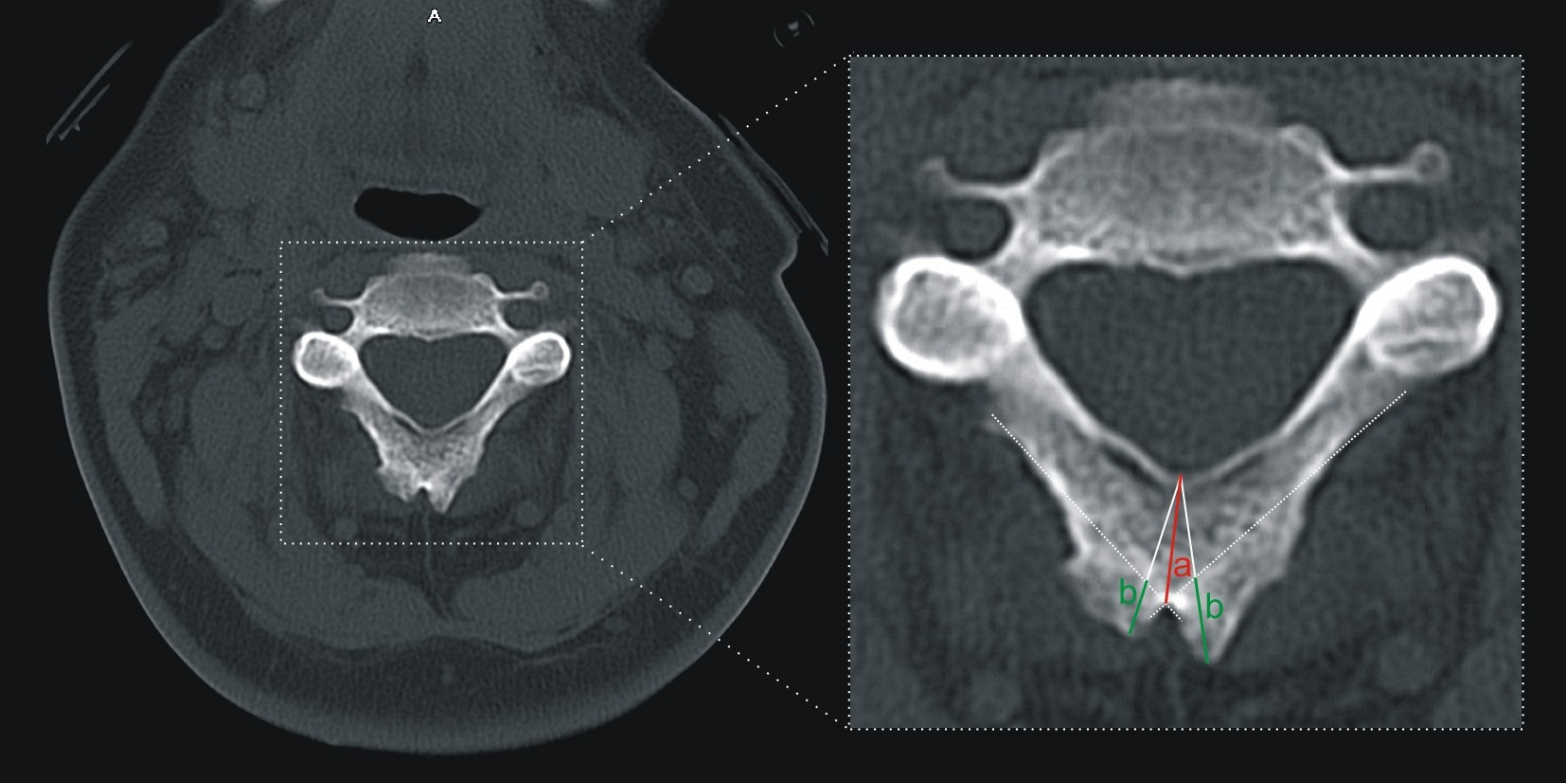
Defined and measured dimensions of the spinous processes. a—length of the spinous process base; b—length of the spinous process branch. Multidetector Computed Tomography (MDCT).
